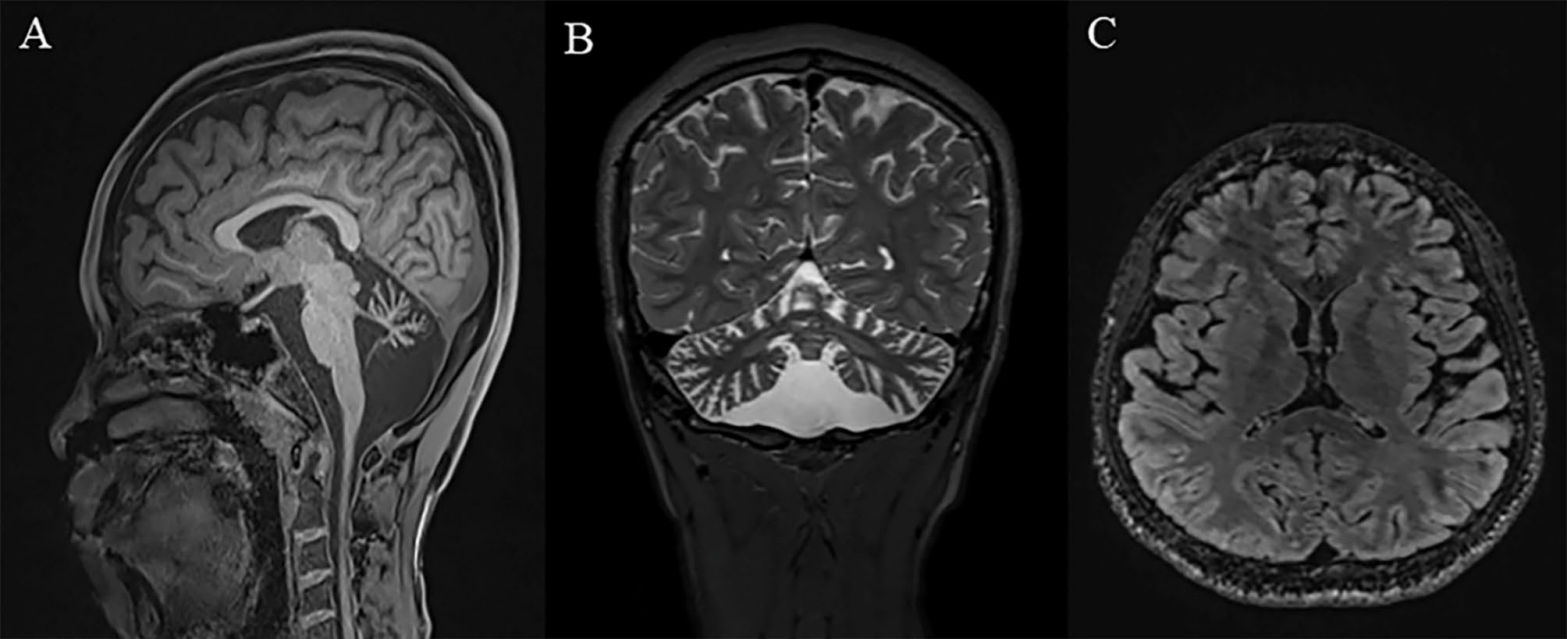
Brain Imaging of this patient. Brain MRI showed severe cerebellar atrophy, enlargement of the fourth ventricle and mild cerebra atrophy. (A - T1 image, B- T2 image). FLAIR imaging showed the basal ganglia was normal (C)
